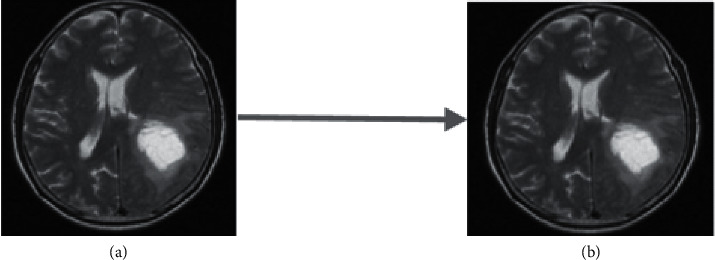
Process of applying median filter in MRI brain image: (a) image with noise and (b) image after noise removal.
